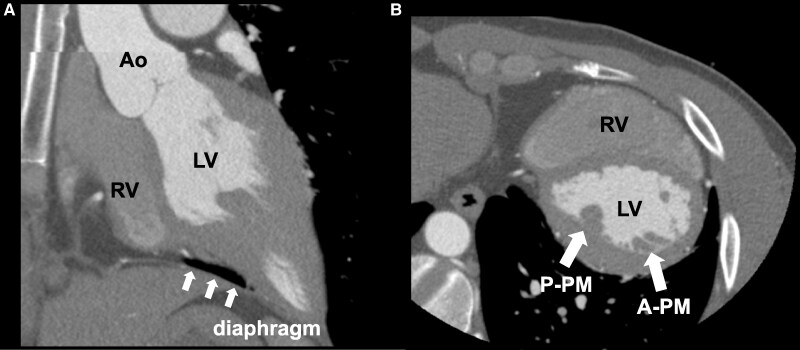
Cardiac multidetector-row computed tomographic images of a 29-year-old man. The right pericardium was partially identifiable; however, this was not confirmed in the left pericardium. Multidetector-row computed tomography shows interposition of the pulmonary tissue between the inferior wall of the LV and the diaphragm (*A*). The anterolateral PM is located posterior to the posteromedial PM because of the significant clockwise rotation of the heart (*B*). A, anterior; Ao, aorta; LV, left ventricle; P, posterior; PM, papillary muscle; RV, right ventricle.
